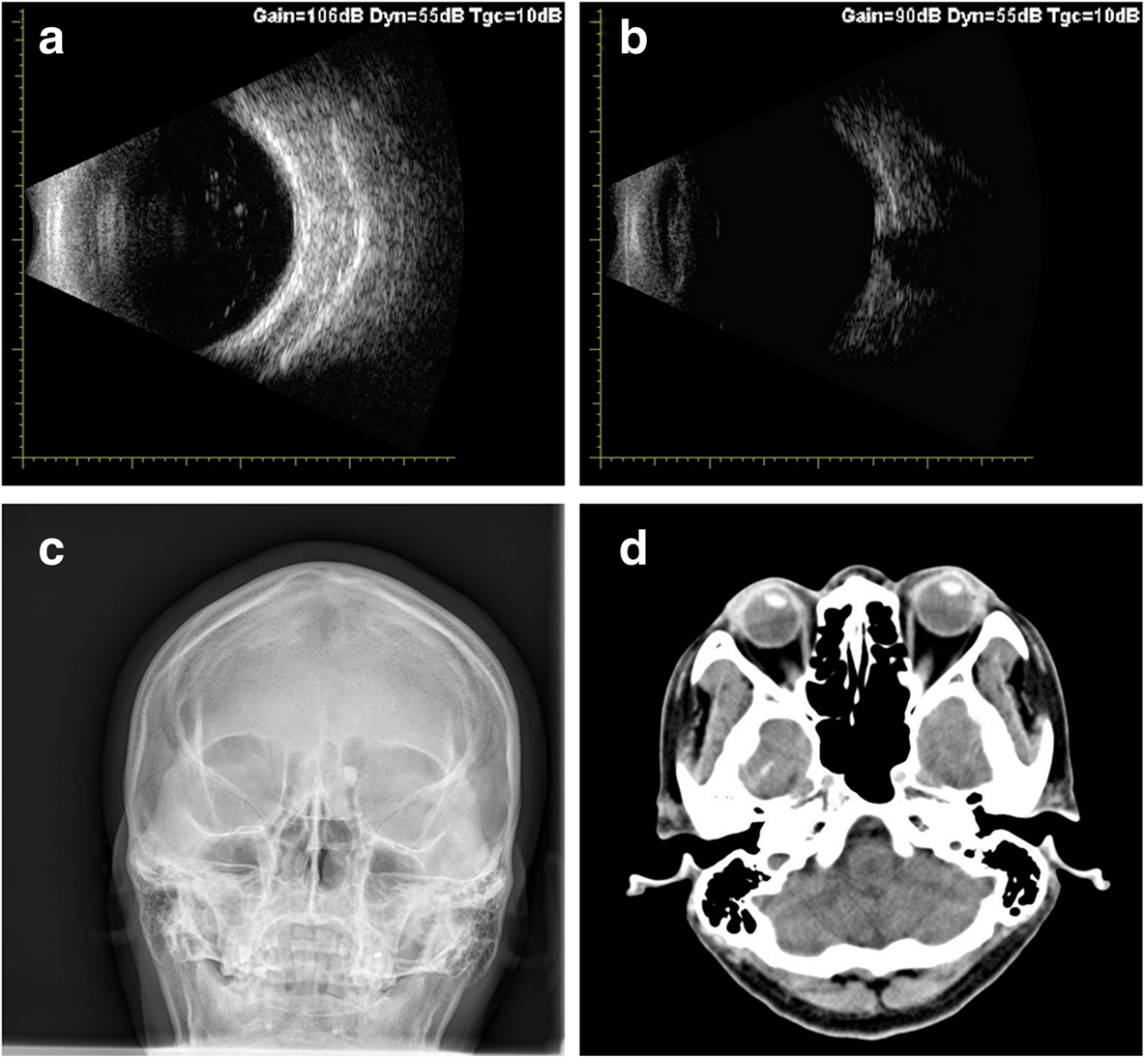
(a-b) Ultrasound B-scan of the posterior segment in low gain settings. The posterior capsule is intact and there is no foreign body seen intraocularly. (c) X-ray of orbits showing no radiopaque foreign body in Water's view. (d) Computed tomography scan showing normal intact globe with no intraocular foreign body in the right eye
